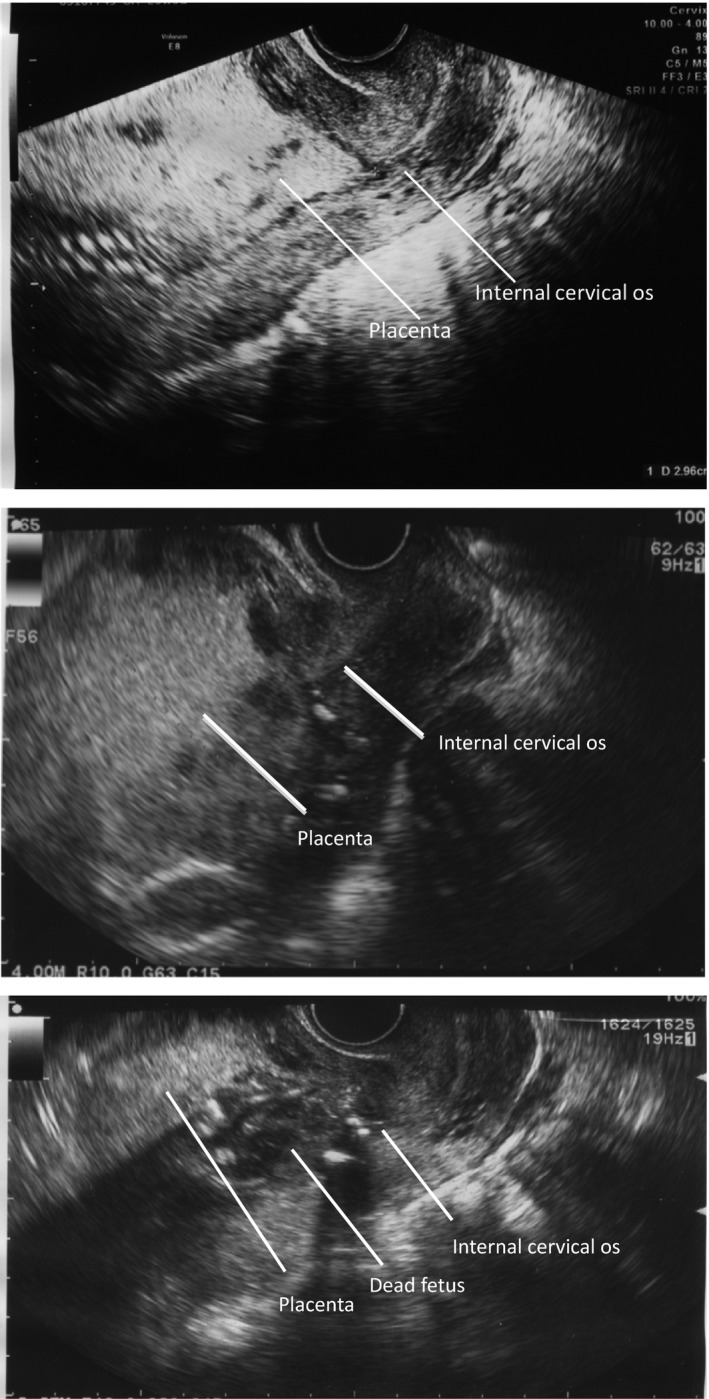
Case 1. Ultrasonographic image taken at 25 weeks and 1 day of gestation. Placenta previa: the placenta completely covers the internal cervical os. Ultrasonographic image taken at 26 weeks and 0 day of gestation. The placenta completely covers the internal cervical os. Ultrasonographic image taken as 28 weeks and 6 days of gestation (22 days after intrauterine fetal death). The placenta is atrophic and no longer covers the internal cervical os.
